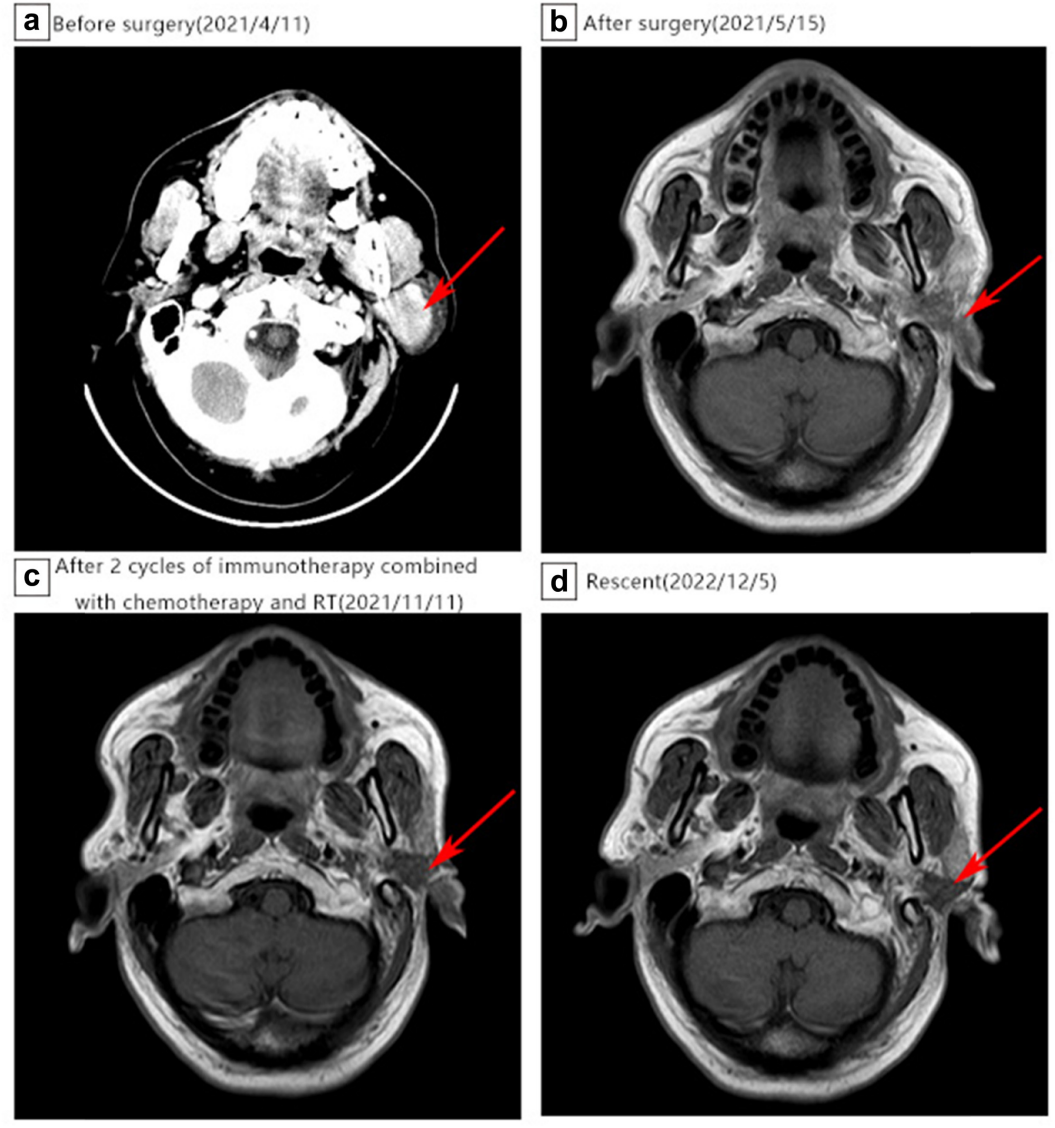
Imaging of the parotid gland. (a) CT scan before surgery, the red arrow shows a left parotid occupancy (on April 11, 2021). (b) Nuclear magnetic resonance (MRI) scan after surgery and before chemotherapy and radiotherapy (RT) (on May 15, 2021). (c) MRI scan after chemotherapy and RT (on November 11, 2021). (d) MRI scan recently (on December 5, 2022).
